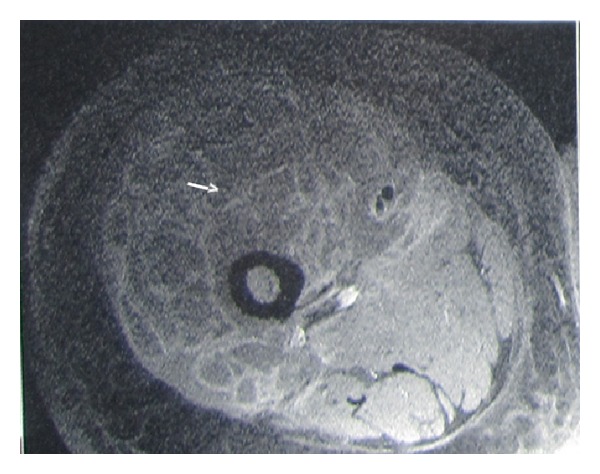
MRI right thigh—MRI axial T1W image—showing a multiseptated intramuscular abscess with debris in quadriceps femoris.
